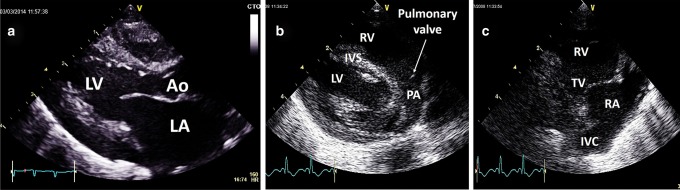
Parasternal long axis view of the LV (a), pulmonary artery (b), and tricuspid valve (c). Ao aorta, LV left ventricle, LA left atrium, IVS inter-ventricular septum, RV right ventricle, TV tricuspid valve, IVC inferior vena cava
